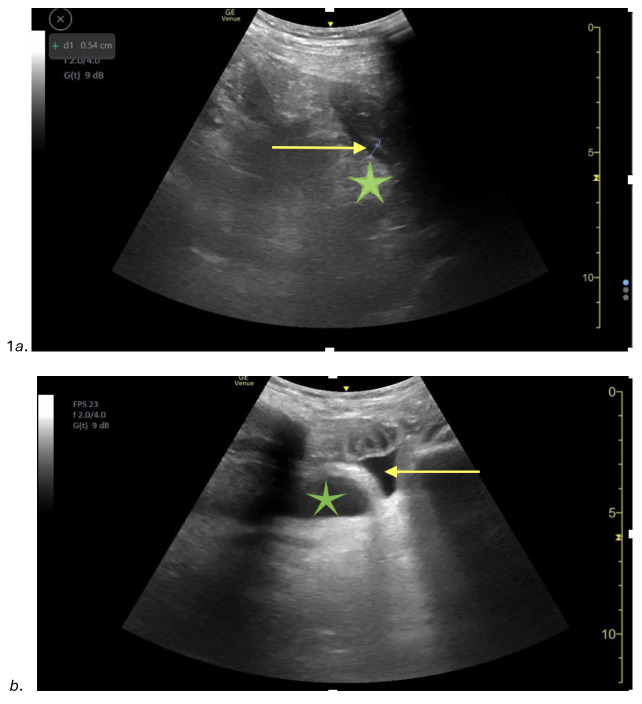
(a): A point of care ultrasound (POCUS) image of the lower abdomen using a curvilinear probe showing a thickened and edematous bowel wall (arrow) and enhancement of the pericolonic fat (star). (b): A point of care ultrasound (POCUS) image of the lower abdomen showing a dilated loop of bowel by the bladder (star) with evidence of free-fluid (arrow) and enhancement of pericolonic fat.
